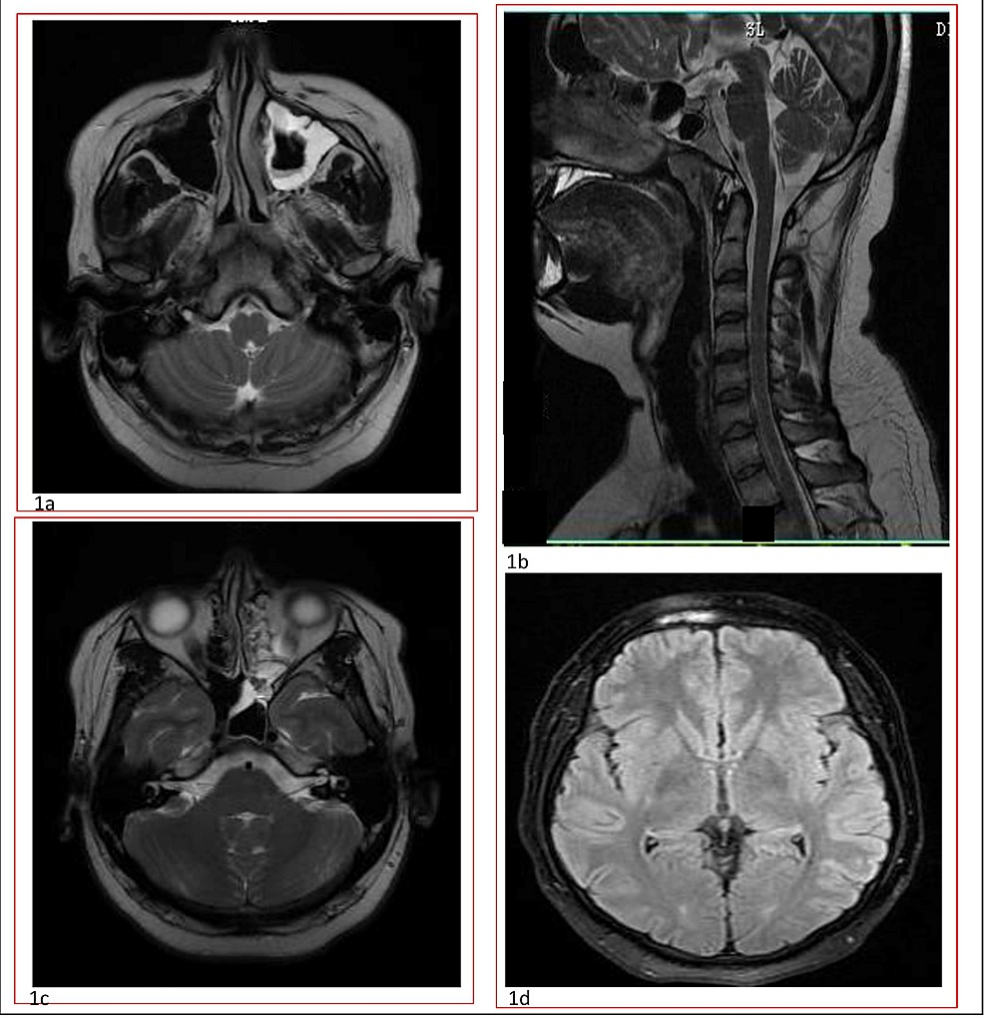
Axial section of T2 MRI brain at the level of the medulla (1a) and pons (1c) showing normal anatomy except deviated nasal septum. Figure (1b) is T2 MRI sagittal section showing the base of the brain where cerebellar tonsils are much above and odontoid process below McRae line indicating no herniation or basilar invagination or atlantoaxial impaction, Even Chamberlain line (line joining back of hard palate to opisthion) noted that tip of dens is below this line. Figure (1d) is the T2 Flair axial section of the brain at the level of the internal capsule and the basal ganglia showing normal anatomy.
